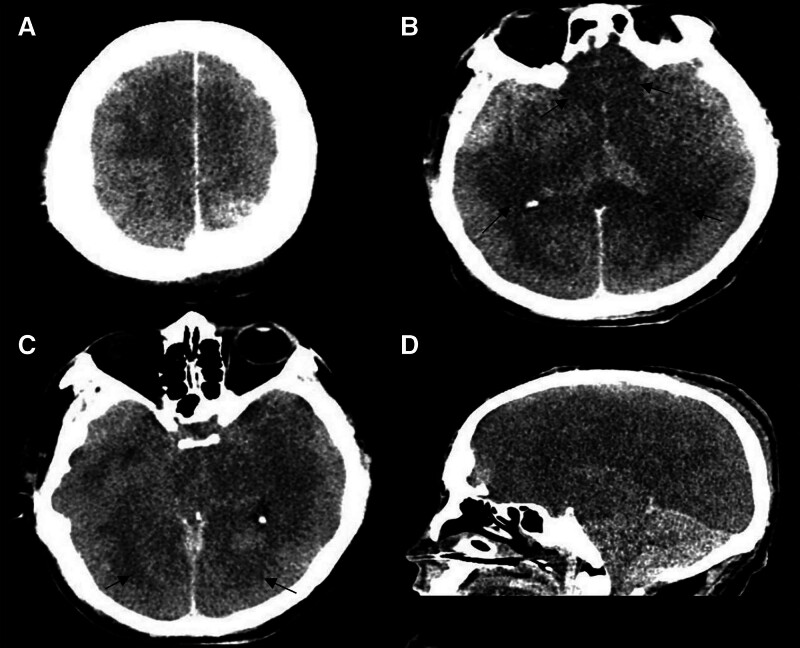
Computed tomography of the head. (A–C) Axial images from the patient’s noncontrast head computed tomography scan, displayed in brain windows. (D) The patient’s cranial computed tomography brain window images in the sagittal view. The black arrow demonstrates symmetric involvement of the brain parenchyma.
